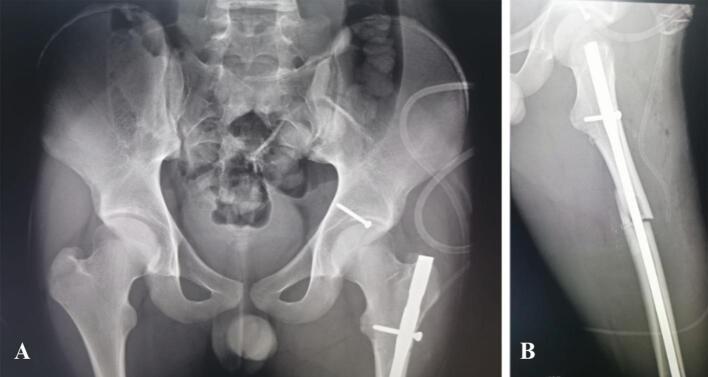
A + B: Post-reduction radiograph showing congruent reduction with a symmetrical widening of the joint space, with a reduction of the femoral shaft fracture.
